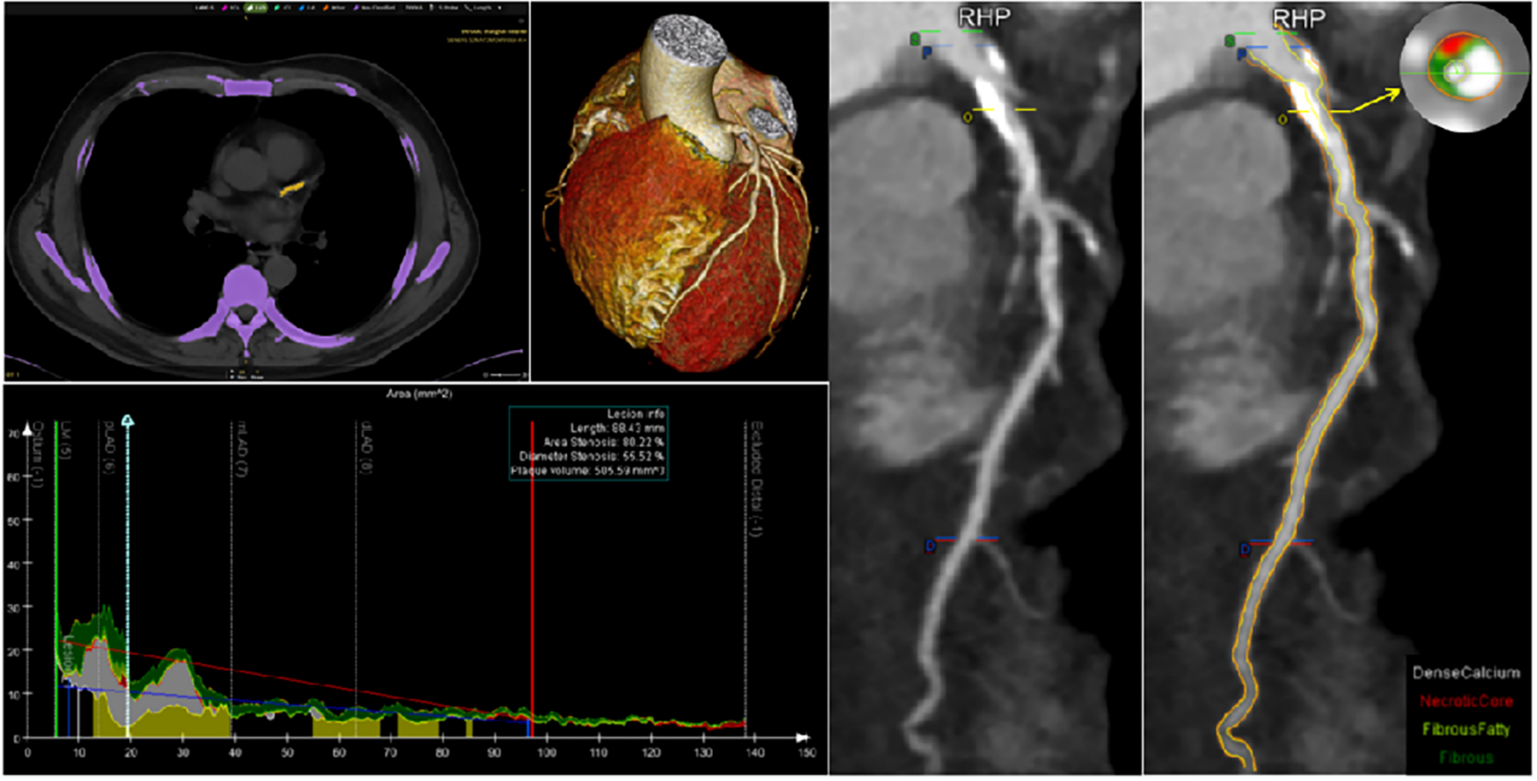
Paradigmatic example of CAC by non-contrast CT, severity of stenosis, and plaque volume analysis by CCTA in left coronary artery. CAC, coronary artery calcium; CCTA, coronary computed tomography angiography; CT, computed tomography.
